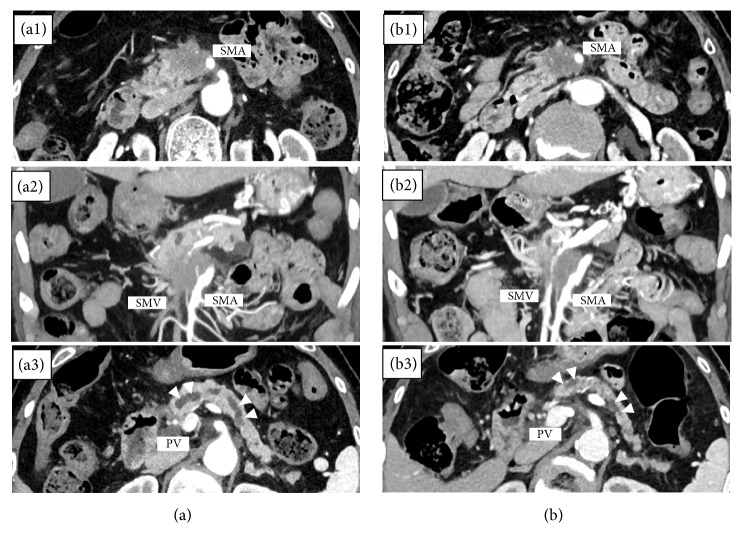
A case of UR-LA PDAC showing drastic reduction of TS, MPDD, and PPV after CRT ((a) before CRT and (b) after CRT. The time interval of CT images between (a) and (b) is 3 months). Arrow head shows main pancreatic duct (a1) and (b1). Cross-section CT images showing the reduction of TS (59.4→52.2 mm) after CRT (a2) and (b2). Coronal section CT images showing the reduction of portal vein invasion after CRT (a3) and (b3). Cross-section CT images showing the reduction of MPDD (7.6→3.7 mm) and PPV (39.8→29.3 ml) after CRT. UR-LA PDAC: unresectable pancreatic ductal adenocarcinoma, TS: tumor size (sum of short and long axis), MPDD: main pancreatic duct diameter, PPV: pancreatic parenchymal volume excluding tumor volume, and CRT: chemoradiotherapy.
